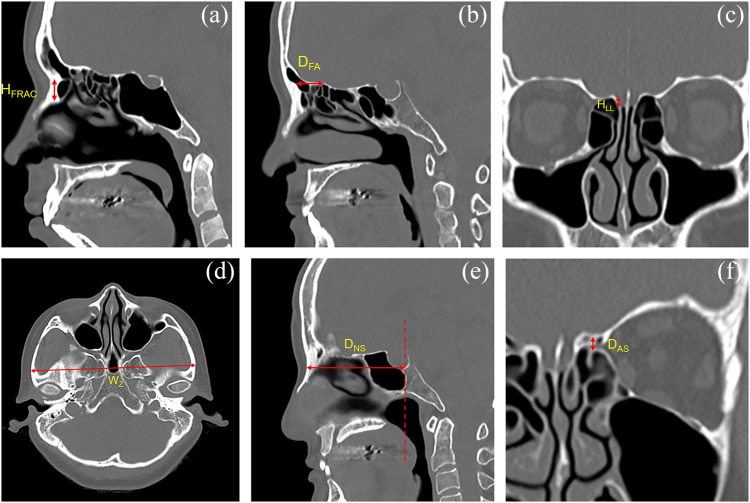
Linear measurements on paranasal sinus computed tomography (CT). (a) Height of the frontal recess anterior cells (H_FRAC_). (b) Frontal beak–anterior ethmoidal artery distance (D_FA_). (c) Height of the cribriform plate lateral lamella (H_LL_). (d) Interzygomatic distance (W_Z_). (e) Nasion–sphenoid distance (D_NS_). (f) Anterior ethmoidal artery–skull base distance (D_AS_).
